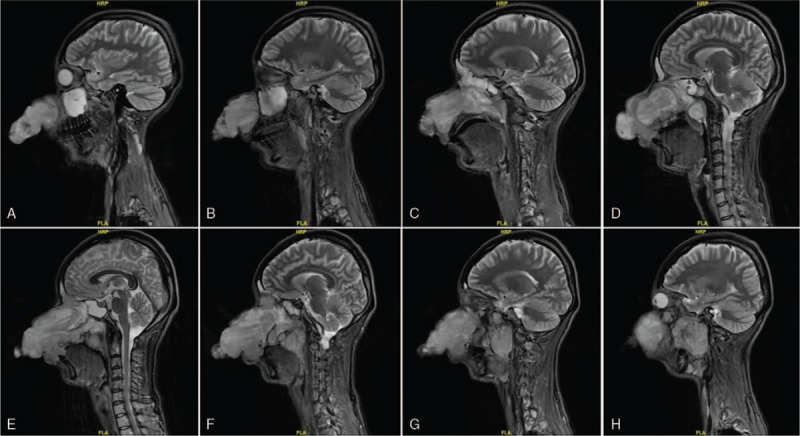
(A–H) Skull sagittal MRI revealed giant occupying lesions with abnormal signals in ethmoid sinus and left infratemporal fossa.
